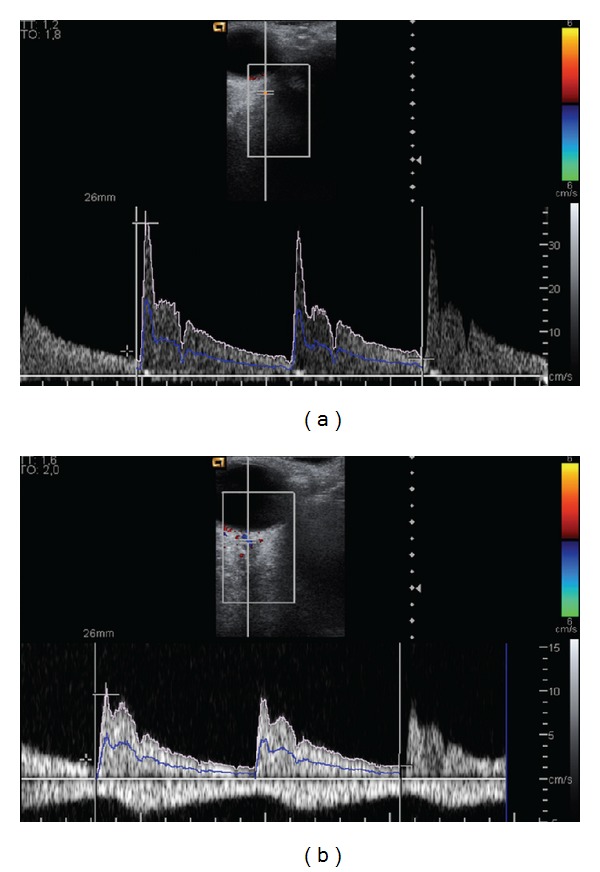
(a) Doppler frequency shift for the ophthalmic artery in the right eye of a patient that showed progression in the HRT-3 (peak systolic velocity PSV = 34.9 cm/s, end-diastolic velocity EDV = 3.7 cm/s, time-averaged velocity TAV = 10.1 cm/s, resistive index RI = 0.89, pulsatility index PI = 3.09, and the relation systole/diastole S/D = 9.43). (b) Doppler frequency shift for the central retinal artery in the right eye of the same patient (PSV: 9.6 cm/s; FDV = 1.4 cm/s; TAV = 3.6 cm/s; S/D = 6.86; RI: 0.85; PI = 2.28).
